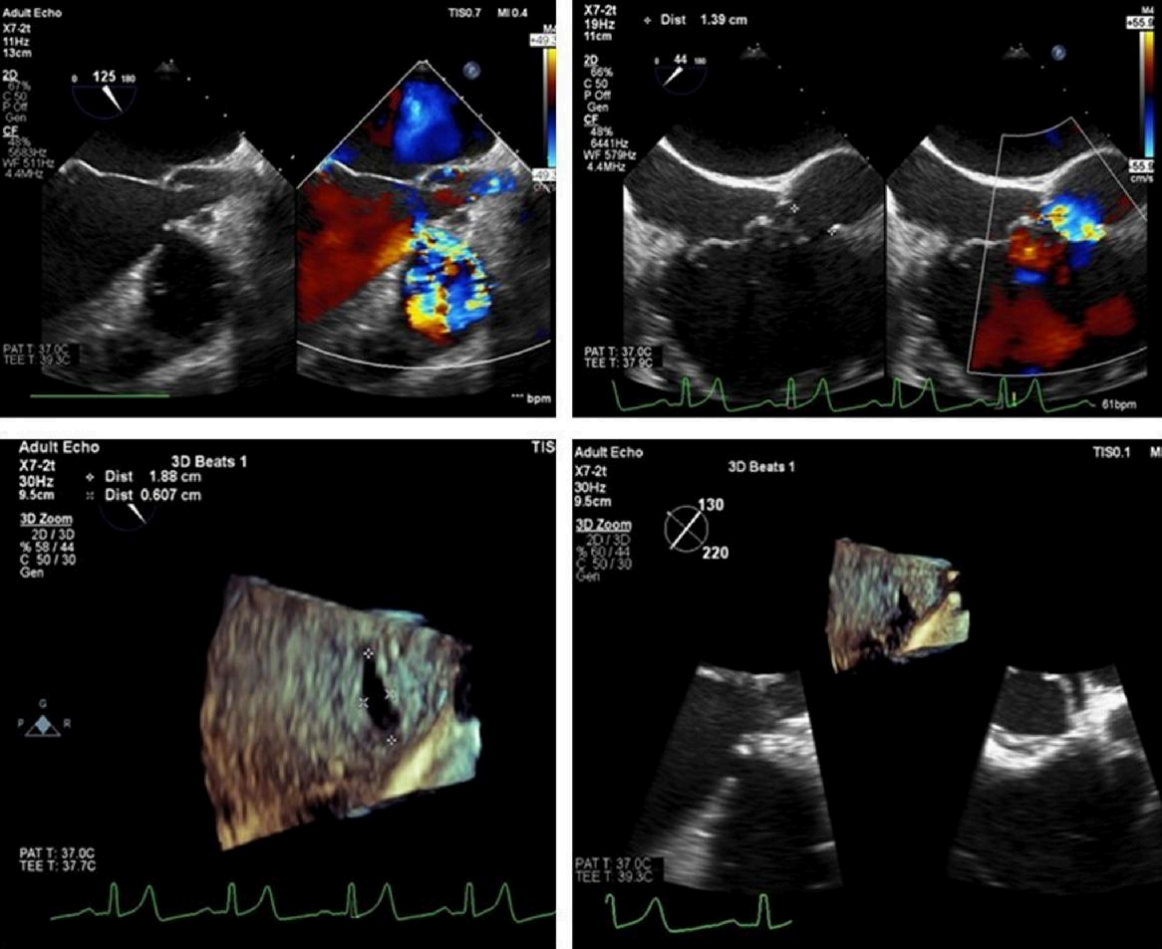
3D echocardiography showing oval-shaped pmVSD with diameter of 18 × 6 mm.
